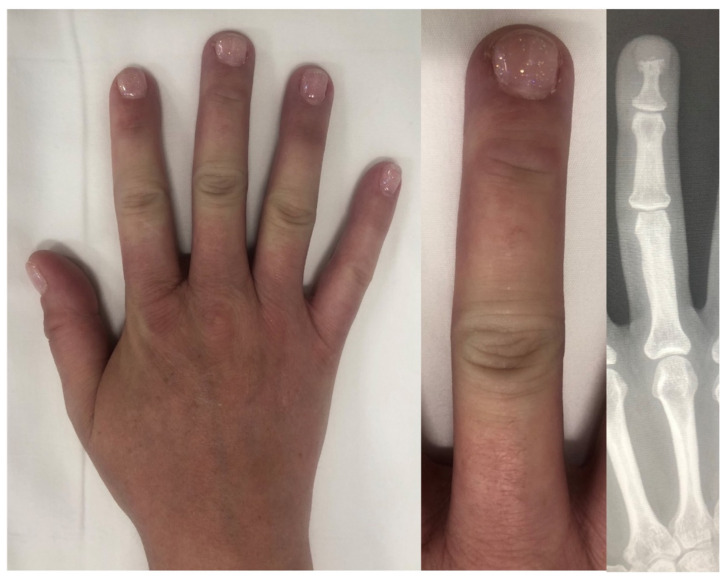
Acro-osteolysis detected on radiographs but not visually seen on physical exam.
